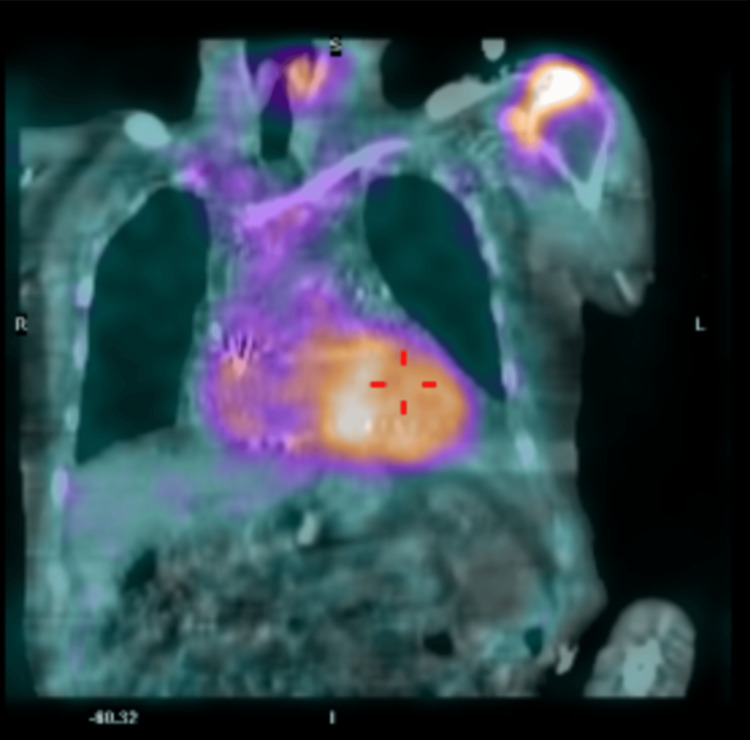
Coronal projection of fused SPECT/CT images displaying intense Tc99PYP tracer uptake in the interventricular septum SPECT/CT images acquired 60 minutes after the injection of radiotracer. SPECT: Single-photon emission computed tomography; Tc99m PYP: technetium pyrophosphate
